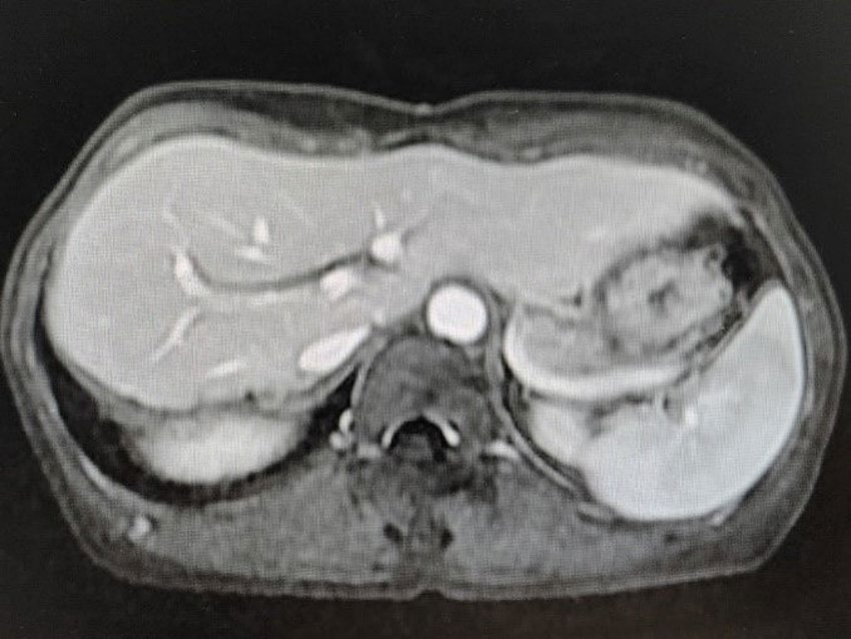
A postoperative MRI scan 8 months later showed no evidence of IMT recurrence.
